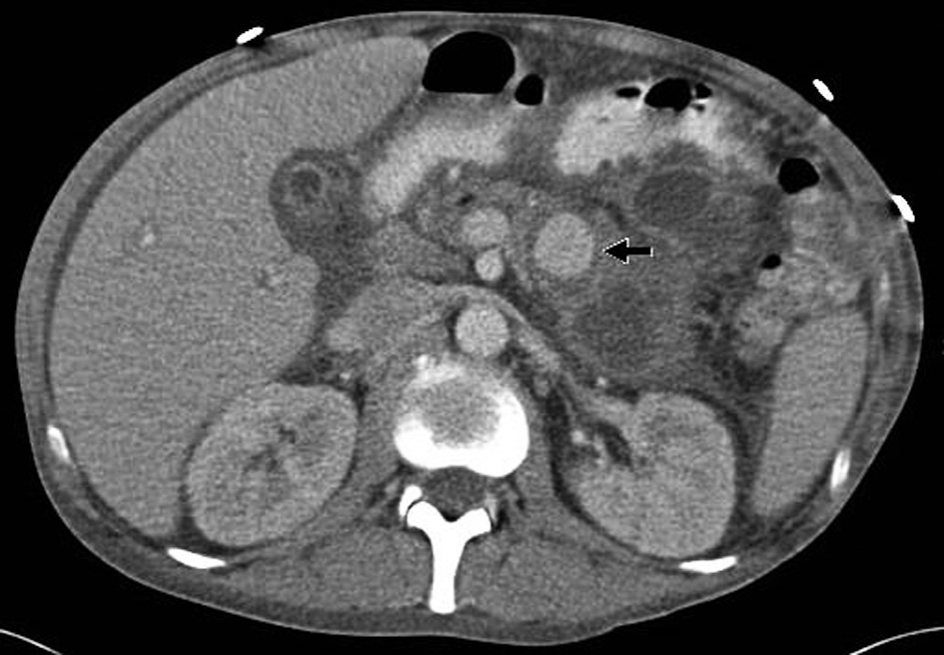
Pre-procedure CTA demonstrating diffuse pancreatitis with pseudocyst and a large pseudoaneurysm arising from a branch of the superior mesenteric artery.
